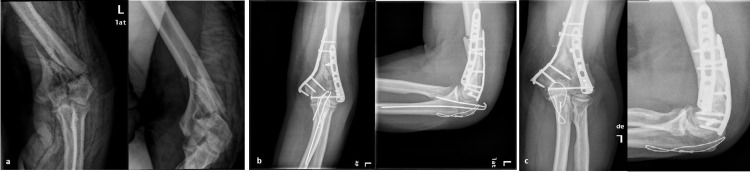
A case of type C intra-articular fracture (a) preoperative anteroposterior and lateral radiograph of an intraarticular fracture of the distal humerus (patient No 6), (b) one-month postoperative radiograph showing adequate reduction with the use of double plating, (c) late follow-up radiograph showing grade 3 post-traumatic arthritis; these patients had also removal of KW under local anesthesia.
